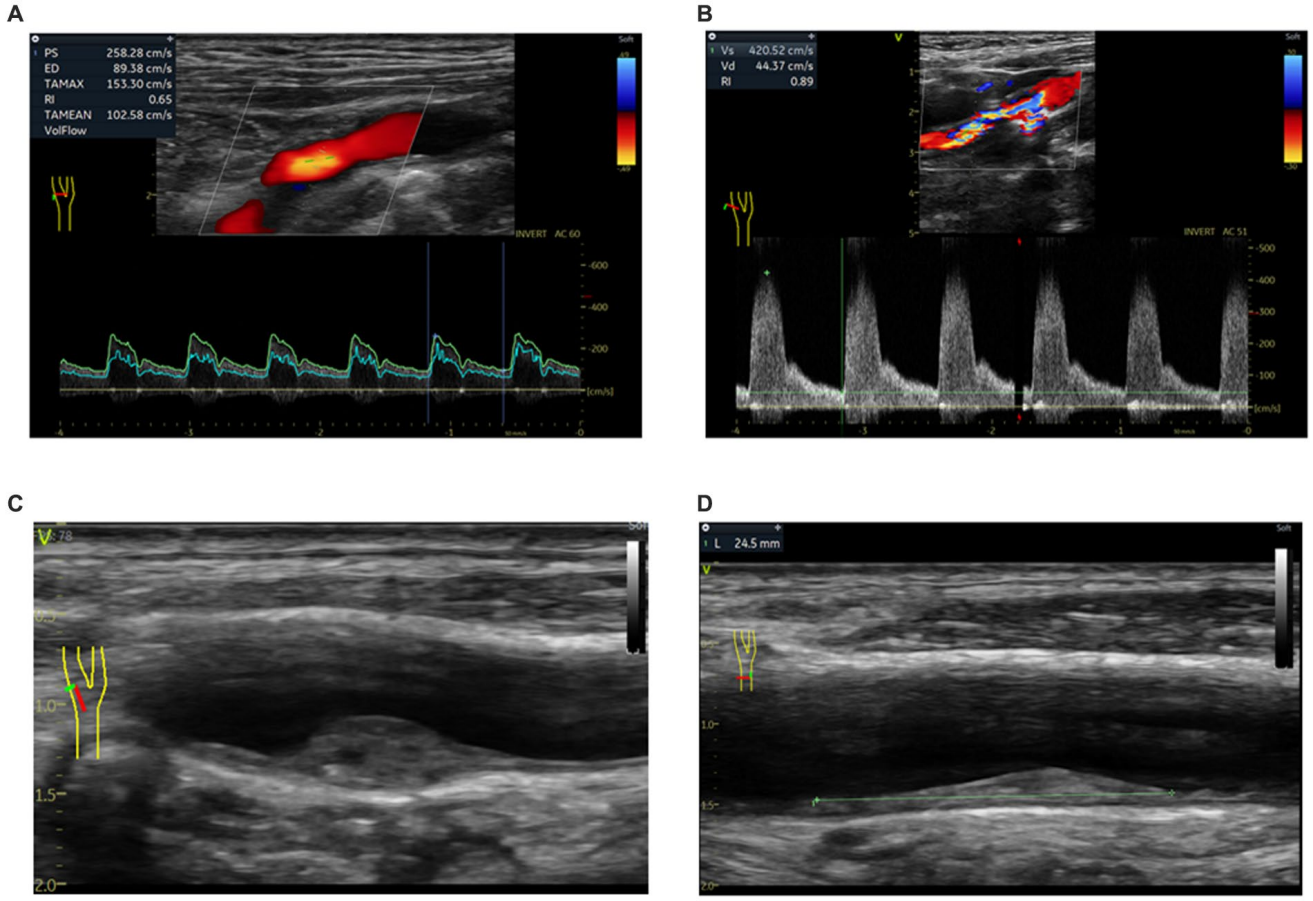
Characteristics of carotid atherosclerosis by carotid ultrasonography. (A) >50–69% stenosis internal carotid artery; (B) > 70% stenosis internal carotid artery; (C) vulnerable plaque in bifurcation; (D) stable plaque in common carotid artery.
